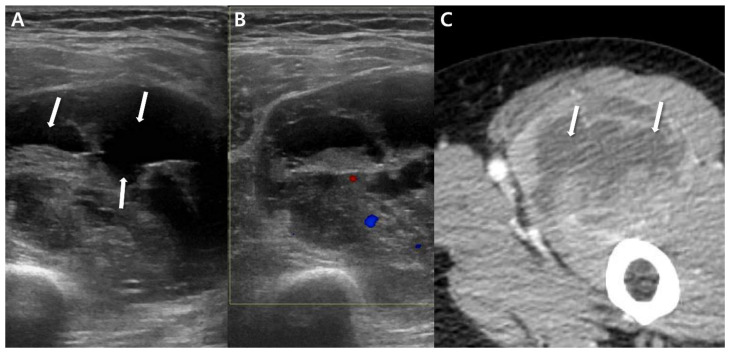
Ultrasonographic features of synovial sarcomas of 63-year-old women (undifferentiated type): (A) Ultrasound shows heterogeneously anechoic and echogenic mass. Anechoic regions (arrows) reflecting cystic/necrotic change. (B) Doppler image shows tumor vascularity. (C) Axial post-contrast CT image shows heterogeneously enhancing mass including non-enhancing portion (arrows) in left thigh.
